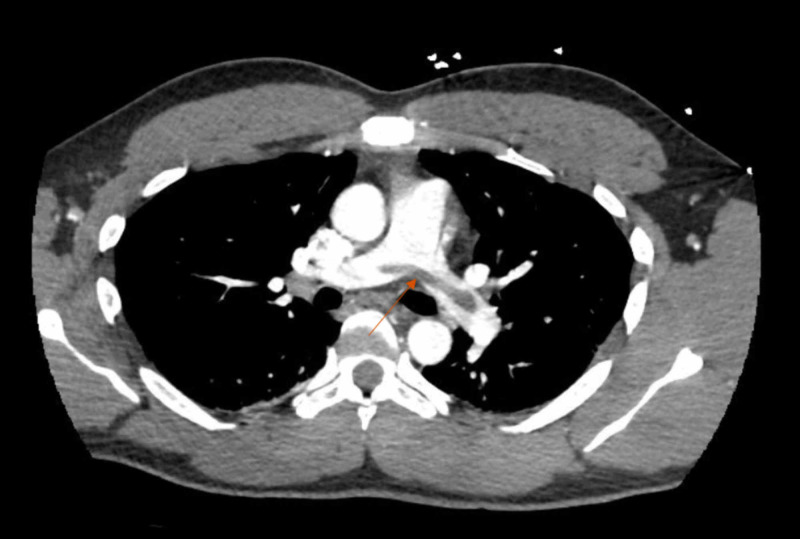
A CT angiography of the chest showing a large saddle pulmonary embolism (arrowhead)
